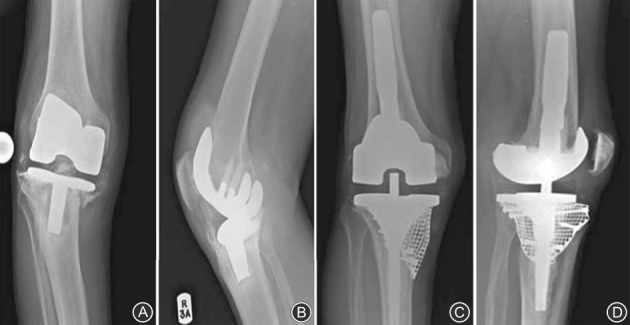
(A, B) Preoperative radiograph anteroposterior view and lateral view of a large tibial bone defects with instability. (C, D) Postoperative radiograph anteroposterior view and lateral view of short cemented stem with impacted bone graft for substantial tibial bone loss.
